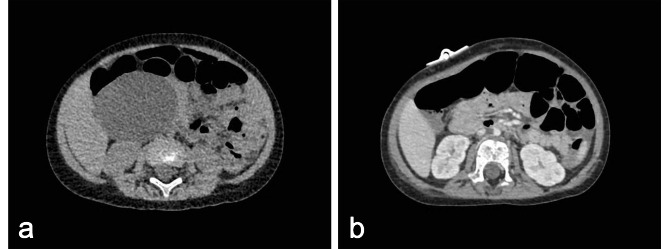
The size of choledochal cyst before and after US-guided percutaneous external drainage. (A) CT before US-guided PBD, Case 4. (B) CT after US-guided PBD, Case 4
